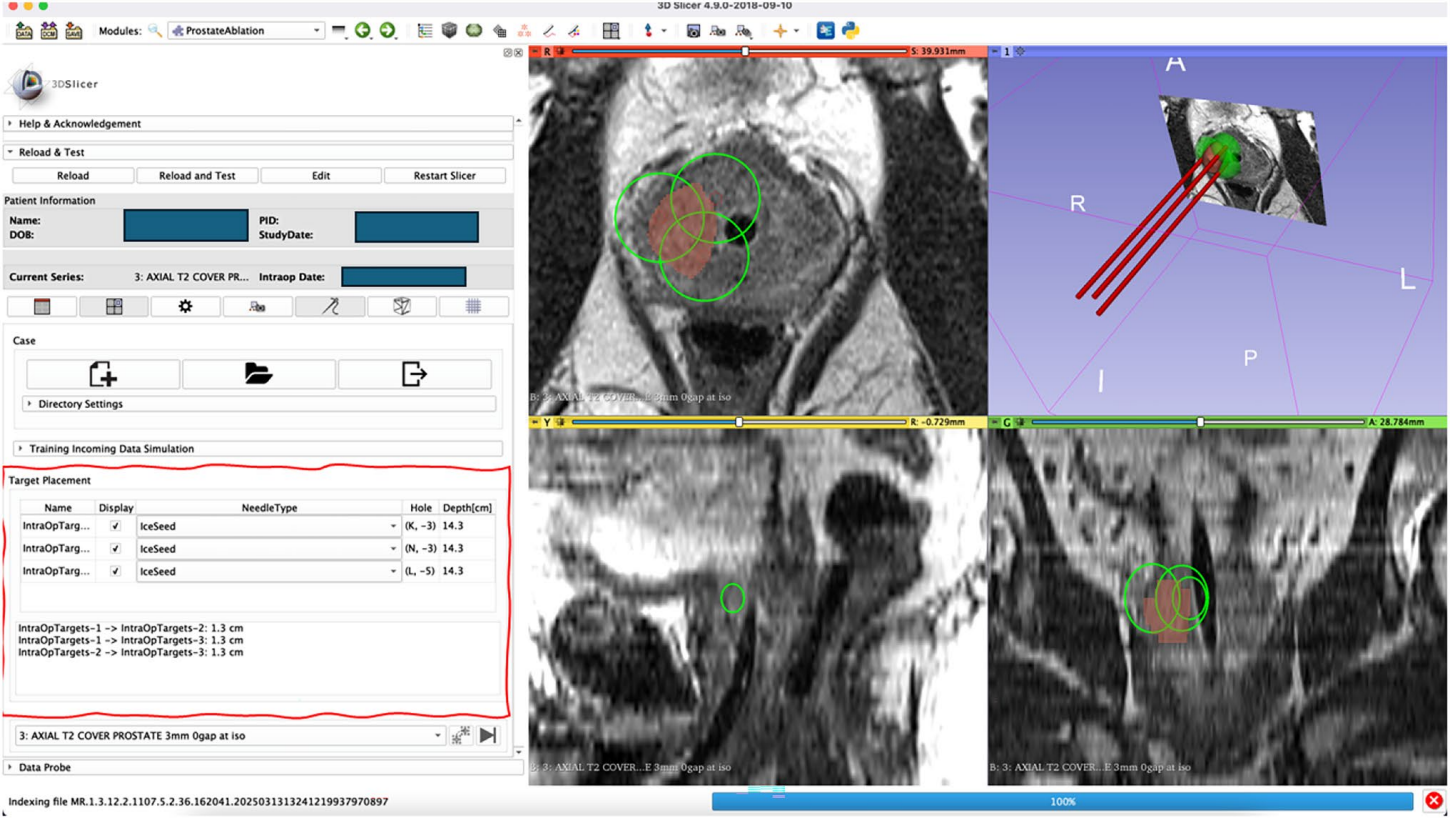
The custom-developed 3D Slicer module for MRI-guided guidance. The physician can define the desired needle placement and the software calculates the template coordinates for each needle to be inserted. The information is provided to the physician on the red-marked area
